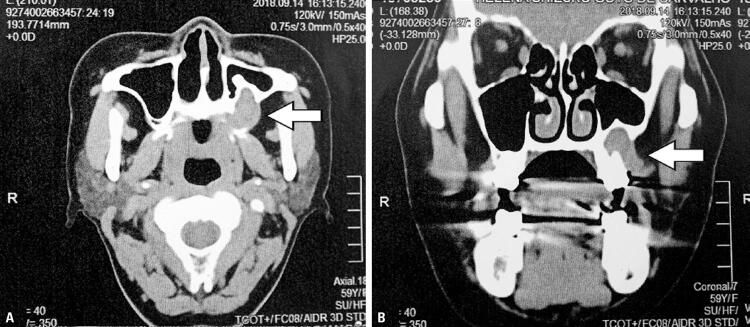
Computed tomography in axial (A) and coronal (B) slices, showing a mass with little enhancement in the left pterygopalatine fossa (white arrows) that was pushing ventrally to the posterior wall of the ipsilateral maxillary sinus, without evidence of bone lysis
